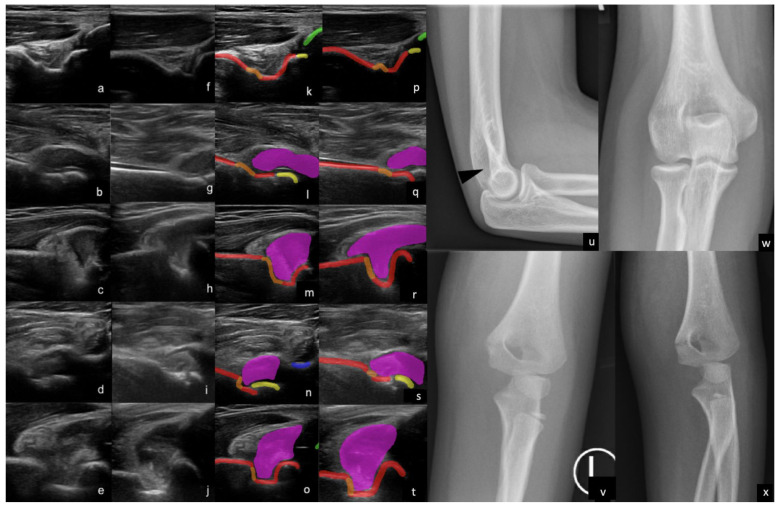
Examples of supracondylar fractures and elbow effusions with anatomy labels superimposed (red = humerus, orange = fracture, yellow = capitellum/trochlea, green = ulna, blue = radius, magenta = effusion). Buckle fracture on a dorsal 2D (a) and 3D (f) US images, with corresponding labels (k,p). Cortical step deformity, effusion, and fat pad elevation representing a fracture on a volar 2D (b) and 3D (g) US image, with corresponding labels (l,q). Sharp step of the olecranon fossa, effusion, and fat pad elevation suggestive of a supracondylar fracture on dorsal 2D (c) and 3D (h) US images, with corresponding labels (m,r). Subtle cortical interruption and effusion representing a minimally displaced fracture on volar 2D (d) and 3D (i) US image, with corresponding labels (n,s). Subtle cortical discontinuity in the superior aspect of the olecranon fossa representing the fracture on dorsal 2D (e) and 3D (j) US images, with corresponding labels (o,t). Radiographs (u,w) corresponding to the 2/3D US images (a,f,k) and (p). Note the subtle contour irregularity in the olecranon fossa cortex (black arrow head), which is suspicious for a buckle fracture and much more readily apparent on US. Radiographs (v,x) corresponding to (b,g,l) and (q) 2/3D US images demonstrating the fracture line. Note the marked soft tissue swelling. This patient was unable to flex their elbow; though an effusion is highly suspected, it is not actually demonstrated on radiographs, but can be easily observed on both volar and dorsal 2/3D US images.
